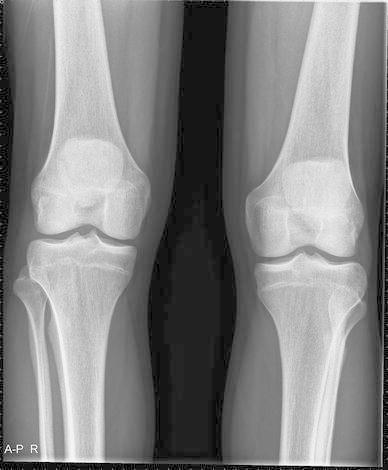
Bilateral post-reduction AP X-ray of the knees showing a reduced fibula on the right side
